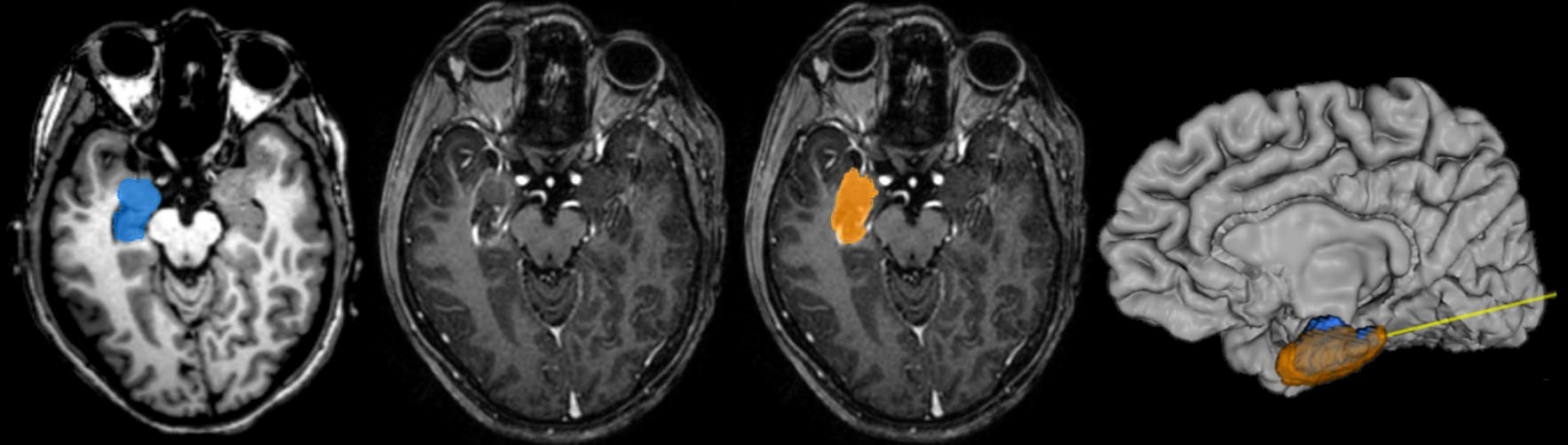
Postoperative MRI in a HS case. Final contrast-MRI sequence and segmentation of the planned (marked in blue) and ablated (marked in orange) volumes with StereoDiver ® for subsequent measurements, considering the internal ring of enhancement, showing the intersection in 3D volume
